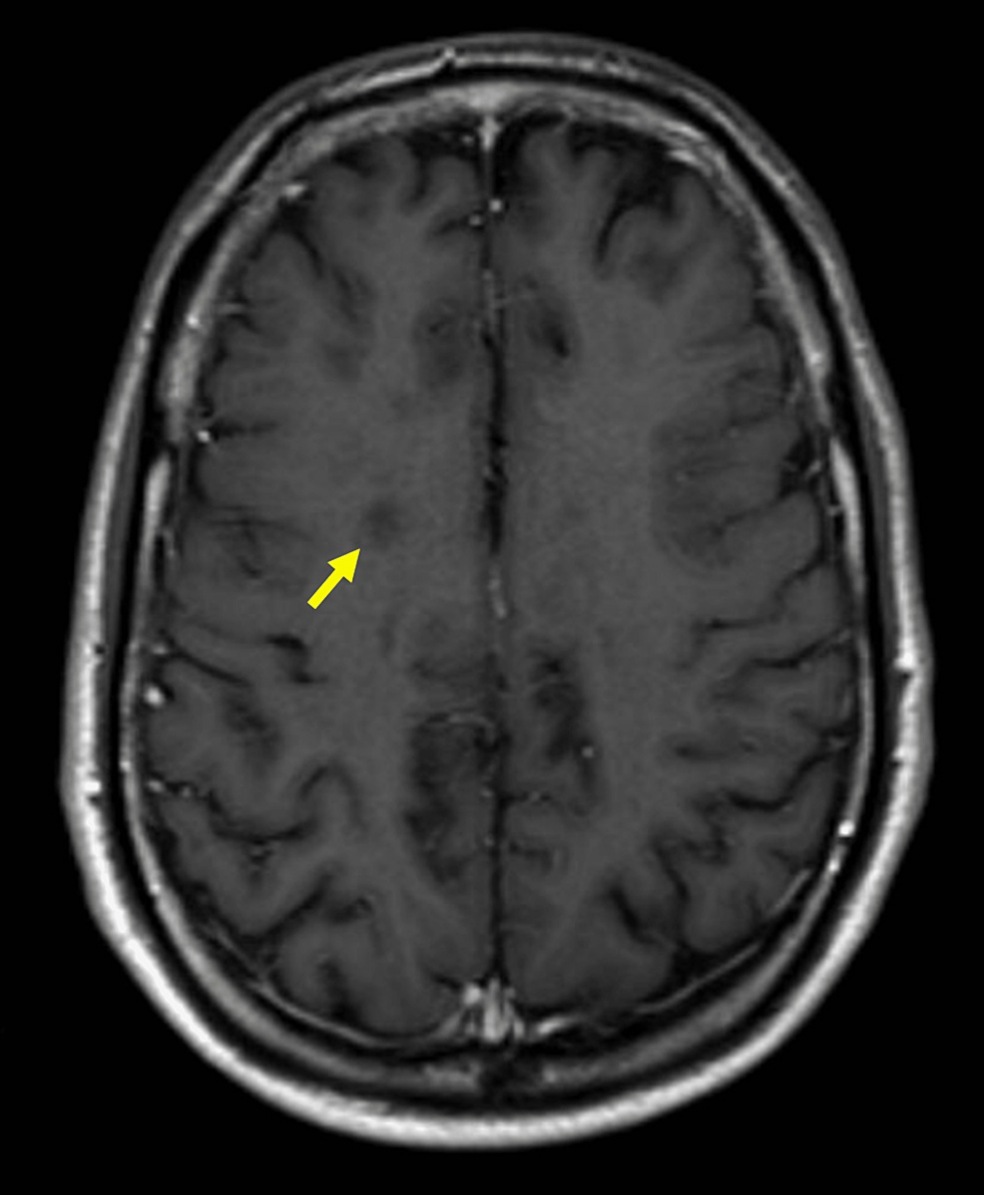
Brain magnetic resonance imaging This image shows hypointense lesions in the right centrum semioval, in T1-weighted image following administration of gadolinium, suggesting lesions with a necrotic center ("black holes" - arrow).
